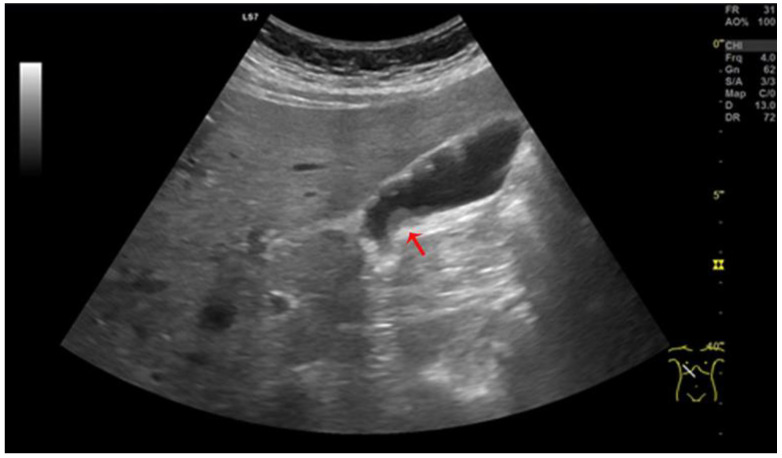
Ultrasonography reveals pancreatic heterotopia Type 1 (red arrow).
